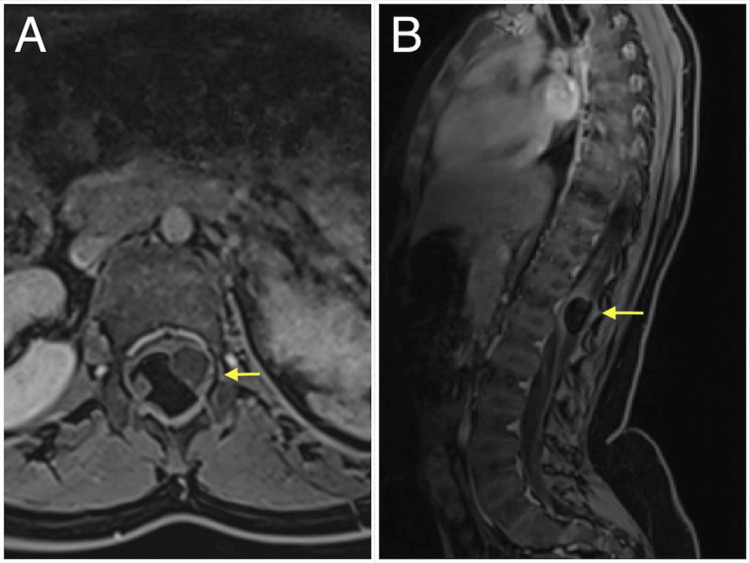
(A) Axial and (B) sagittal post-contrast, fat-suppressed, T1-weighted MRI scans showing a marked hypo-signal of fat component with mild peripheral enhancement (yellow arrow).
